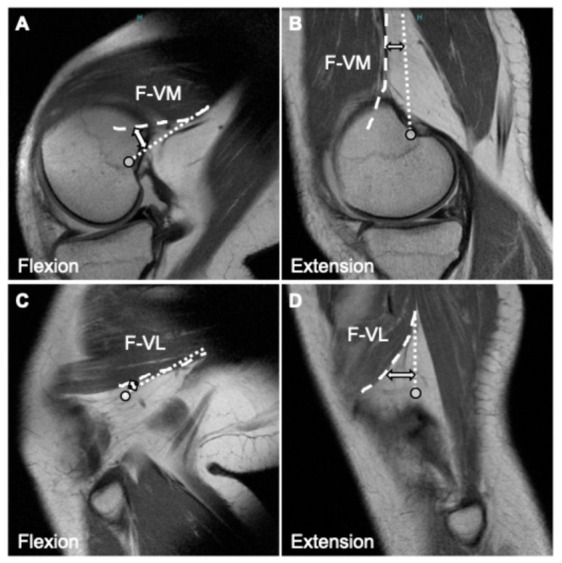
Representative sagittal magnetic resonance imaging (MRI) demonstrating the dynamic positional changes in the vastus medialis (VM) and vastus lateralis (VL) relative to the femur between 10° and 90° knee-flexed positions. (A,B) The distance from the posteromedial femoral cortex to the posterior border of the VM (F-VM): A representative example in the flexion (A) and extension (B) positions. (C,D) The distance from the posteromedial femoral cortex to the posterior border of the VL (F-VL): A representative example in the flexion (C) and extension (D) positions. The double-headed arrows indicate the measurement direction, representing the shortest perpendicular distance from the femoral bone cortex (dotted lines; posteromedial in (A,B) and posterolateral in (C,D)) to the posterior border of the respective muscles (dashed line; VM in (A,B) and VL in (C,D)), measured perpendicular to the longitudinal axis of the femur. As visually demonstrated in these representative images, the quantitative analysis confirmed that the F-VM and F-VL were significantly greater in the extension position compared to the flexion position across all measured levels (0–4 cm). The circle markers represent the predefined reference points (0 cm) consistent with the definitions in Figure 1. The circle markers indicate the zero reference points (0 cm) located at the intersections of the intercondylar line (Blumensaat’s line) and the respective posterior femoral cortices.
